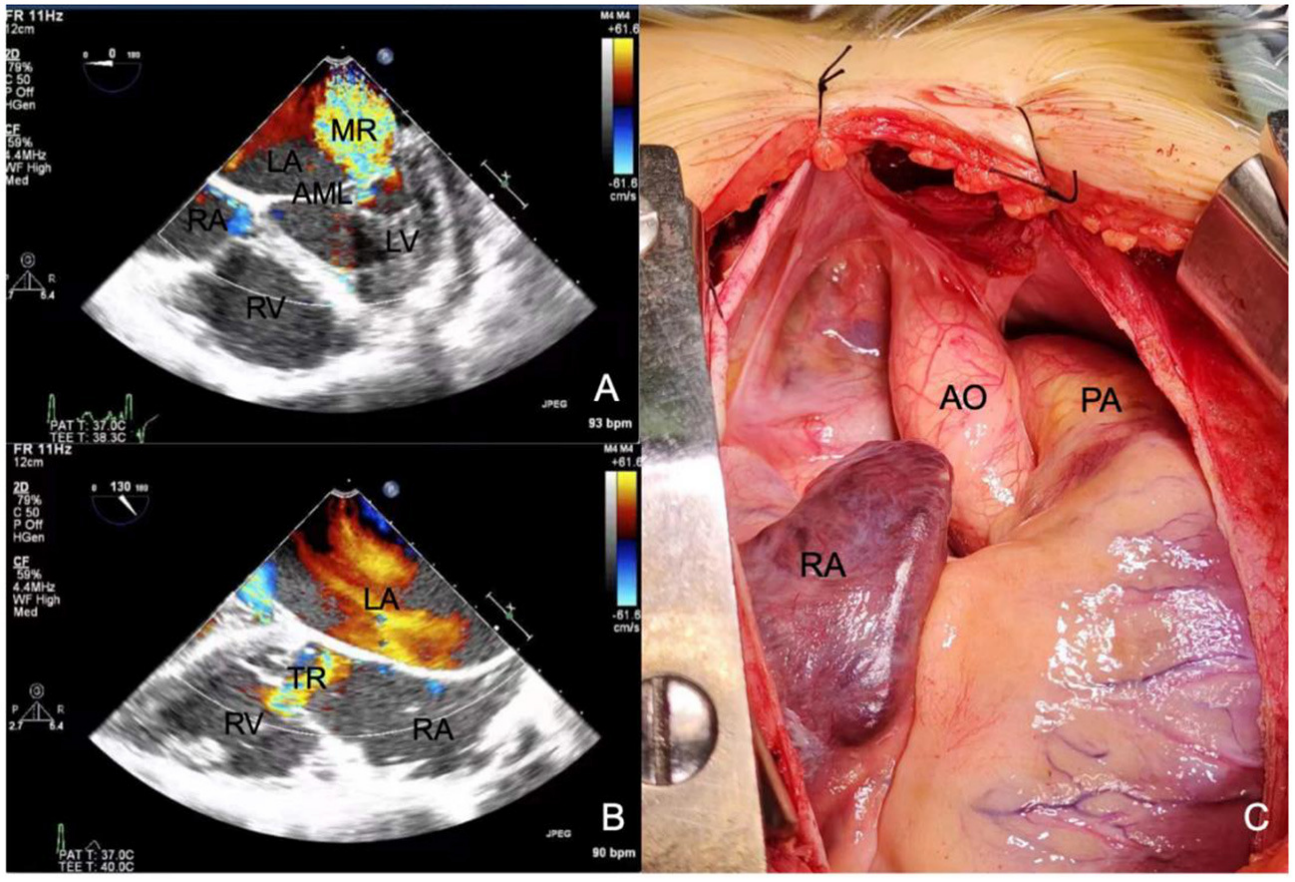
Echocardiography shows (A) severe mitral regurgitation and (B) severe tricuspid regurgitation. (C) Intraoperative photographs show that the aorta is 1.5 cm in diameter, which is significantly smaller than normal. RA, right atrium; RV, right ventricle; LA, left atrium; LV, left ventricle; AML, anterior mitral leaflet; MR, mitral regurgitation; TR, tricuspid regurgitation; AO, aorta; PA, pulmonary artery.
